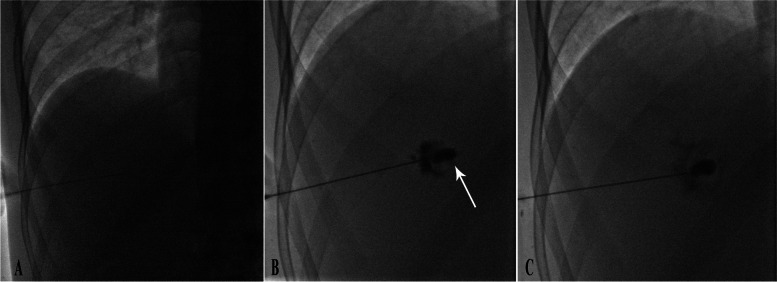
A The lesion is punctured under ultrasound and fluoroscopy guidance. B Contrast injection shows the needle tip in a central cystic area (arrow). Note that the contrast remains in this area and does not spread inside the haemangioma. C The needle is slightly withdrawn, now showing further dilution and dissemination of the contrast agent
